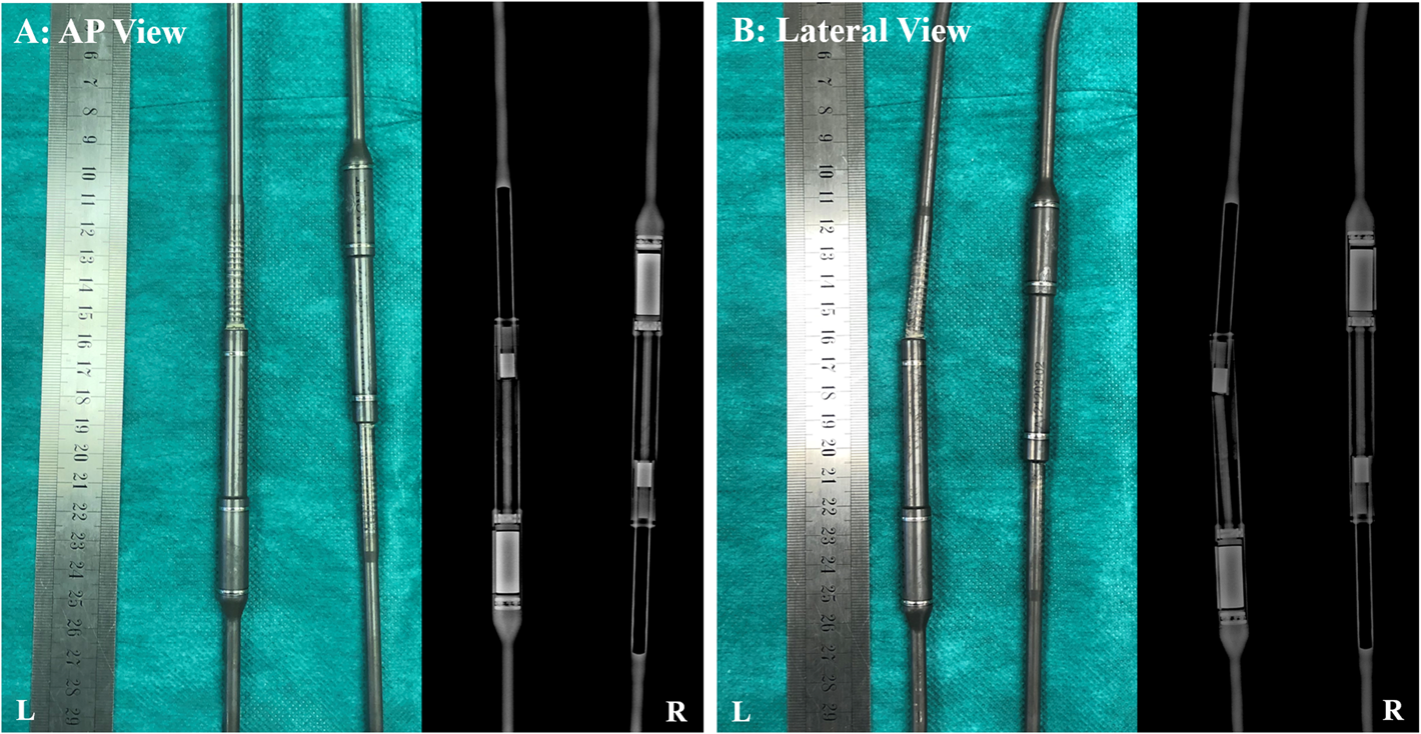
External examination of the final extracted MCGRs. a Both rods were aligned externally and under X-ray investigation in the AP view but some transverse wear marks could be seen on the piston rod portion. b On the lateral view, the “Crooked rod sign” was seen
